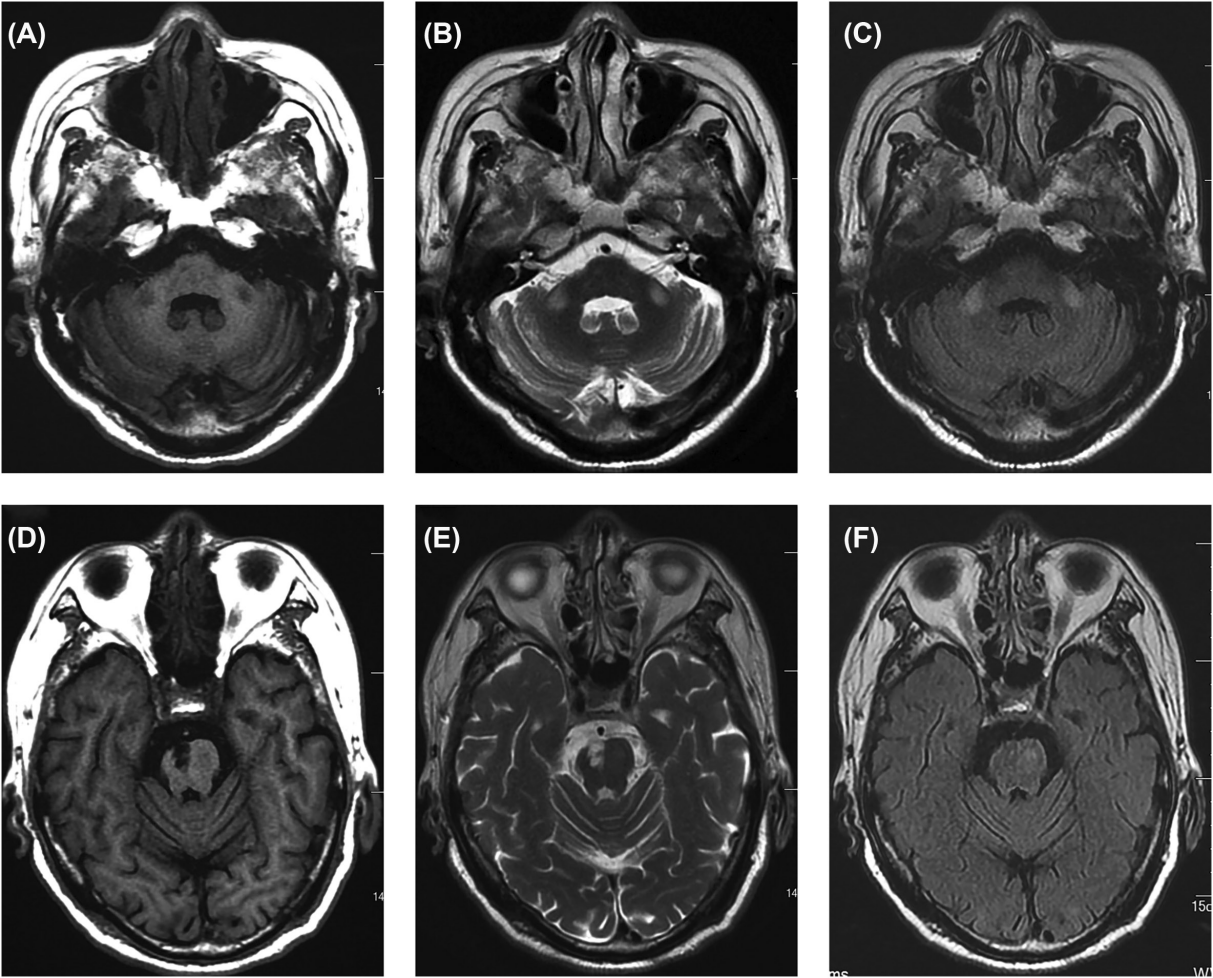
Bilateral symmetrical wallerian degeneration in the middle cerebellar peduncles was observed through MRI. (A–C) symmetrically low T1‐weighted (Axial T1W) signal in the bilateral middle cerebellar peduncles, accompanied by high T2‐weighted (Axial T2W) and fluid‐attenuated inversion recovery (Axial FLAIR) signal intensities. (D–F) low T1W signal intensities in the right pons paramedian, along with high T2W and FLAIR signal intensities, consistent with gliosis/chronic ischemia.
